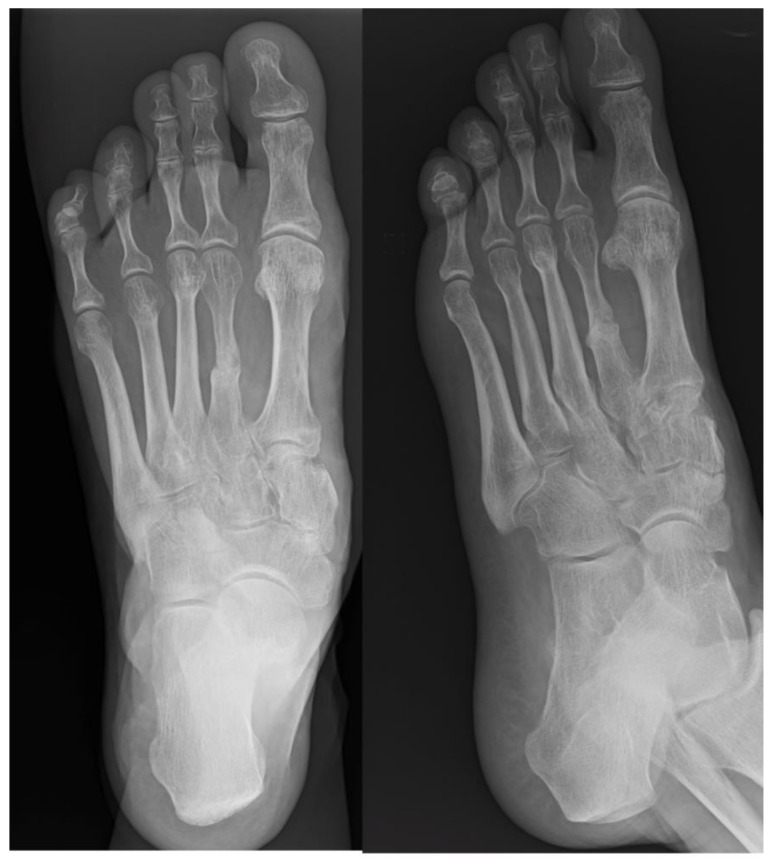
Postoperative weight-bearing X-rays at 8 months showing a solid and well-aligned union of the second metatarsal and restoration of the correct metatarsal formula.
